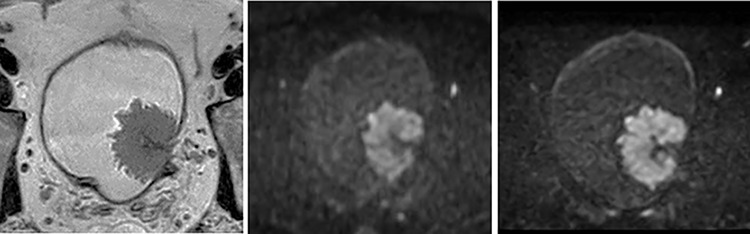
83-year-old woman with the urothelial carcinoma of pT1 on the left lateral wall of the bladder. (A) T2WI (B) fFOV DWI (C) Zoomed DWI. The tumor appeared as a papillary lesion with tumor stalk in both fFOV and Zoomed DWI, and VI-RADS score was 2 for both series.
